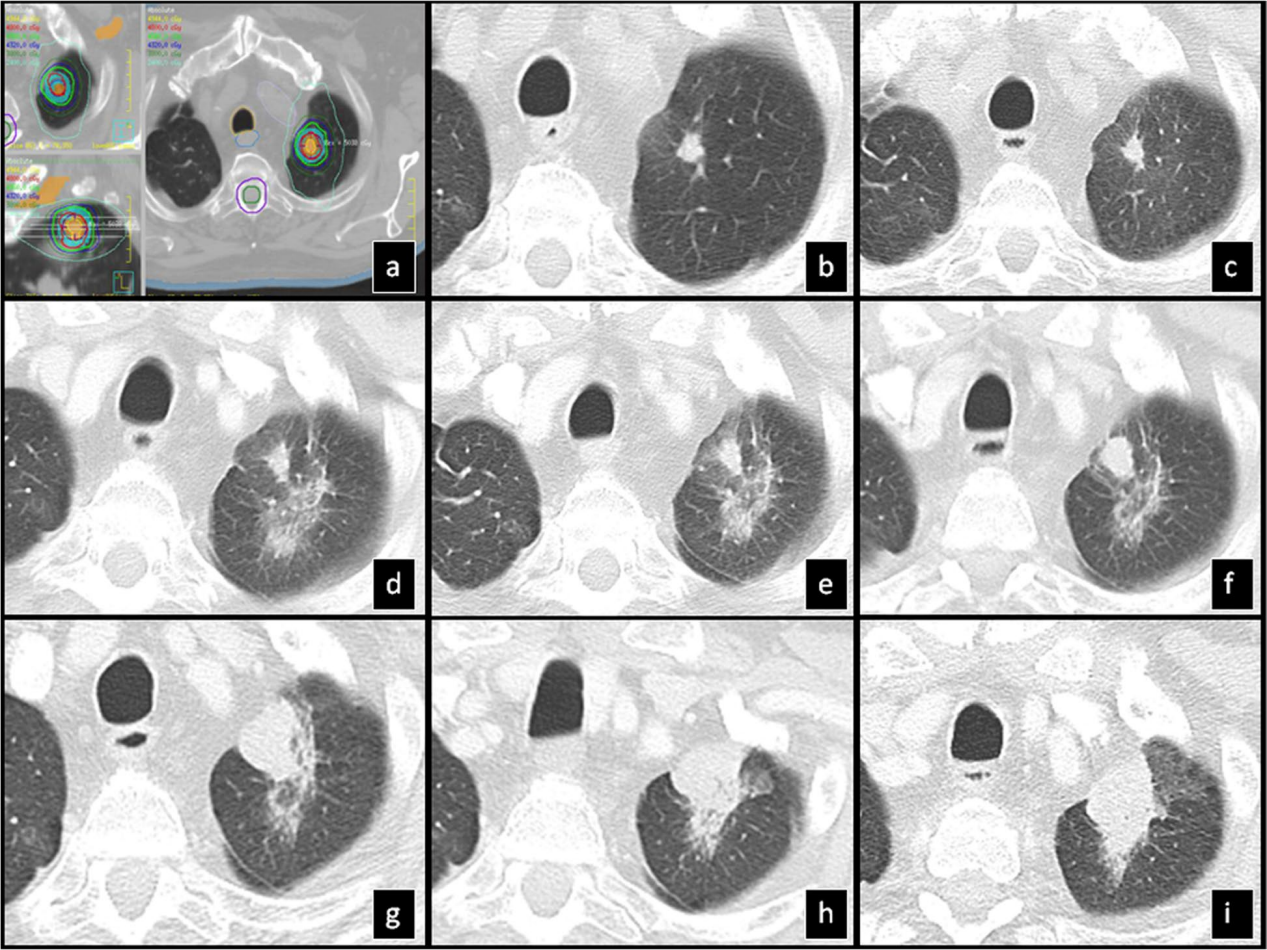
SBRT-treated lung cancer demonstrates local recurrence at 35 months: a) Isodose multiplanar images for planning. b) pre-SBRT CT shows a solid nodule. c) 8 mo post-SBRT, the nodule has not changed significantly in size, however there is new surrounding GGO. d) 13 mo post-SBRT the lesion is stable and there is surrounding patchy GGO and peribronchial consolidation. e) 17 mo post-SBRT, there is slight increase of the nodule size with stable patchy GGO and consolidation. f) 26 mo post-SBRT, there is further mild interval increase of the nodule size and slight improvement of the surrounding GGO and consolidation. g) 31 mo post-SBRT, there is further interval increase of the lesion size (now a mass) and increased density of the adjacent radiation changes. h) 35 mo post-SBRT there is further increase of the primary lesion partially obscured by the surrounding radiation changes which demonstrate increased density. Local recurrence was established at this point. i) 39 mo post-SBRT, there is further increase of the primary lesion which is now inseparable for surrounding radiation changes which demonstrate further increased density
